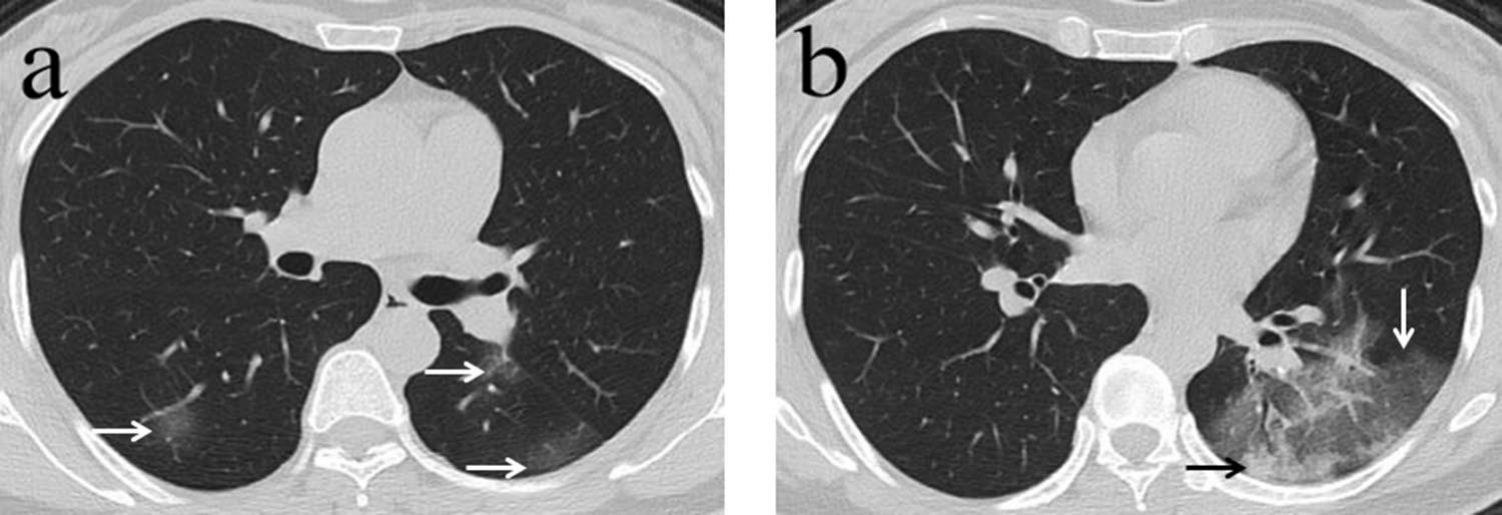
A 45-year-old woman with history of recent travel to Wuhan presented with fever for 1 day. (a,b) Non-contrast enhanced chest computed tomography (CT) showed multiple peripheral patchy ground glass opacities in both lower lobes (white arrows). CT scan also demonstrated consolidation in the left lower lobe (black arrow). CT involvement score was 5.
